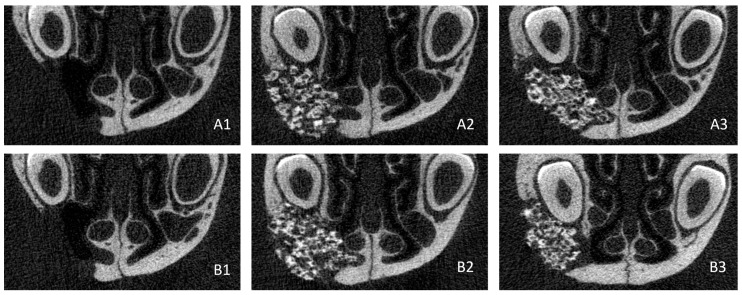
Transaxial CT sections at (A) week 4 and (B) week 8 under the following conditions: (1) empty, (2) biomaterial only and (3) biomaterial + MSCs.
